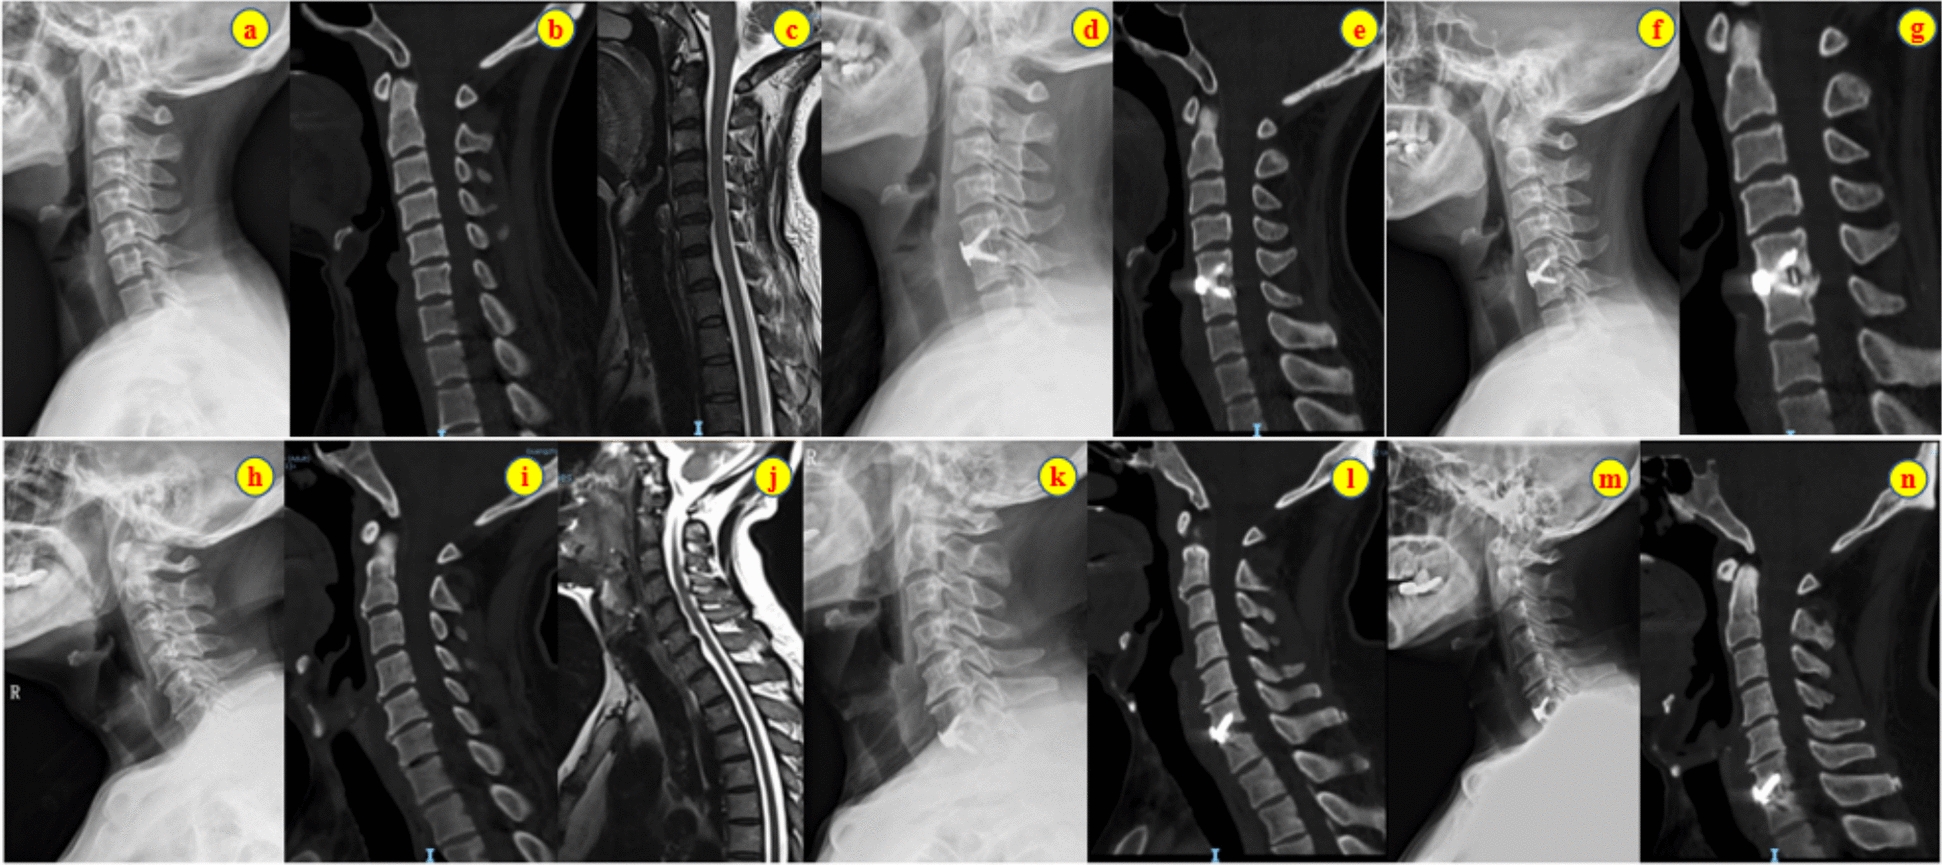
a–g Case of an elderly female patient without sarcopenia who underwent C5/6 ACDF surgery. a–c Preoperative *X*-ray, CT, and MRI examinations revealed cervical kyphosis and left-sided intervertebral foramen-type herniation at the C5/6 level; d, e Immediate postoperative *X*-ray and CT scans indicated stable fixation of the C5/6 internal fixation; f, g Two-year postoperative *X*-ray and CT scans revealed robust fusion at the C5/6 level. At 2-year postoperative, her symptoms improved significantly, with minimal discomfort. h–n Case of an elderly male patient with sarcopenia who underwent C6/7 ACDF surgery. h–j Preoperative examination revealed C6/7 intervertebral disc herniation posteriorly and centrally. k, l Immediate postoperative *X*-ray and CT scans revealed stable fixation of C6/7 internal fixation. m, n 2-year postoperative *X*-ray and CT scans indicated strong fusion at C6/7. Follow-up revealed residual radicular symptoms 2 years postoperatively
